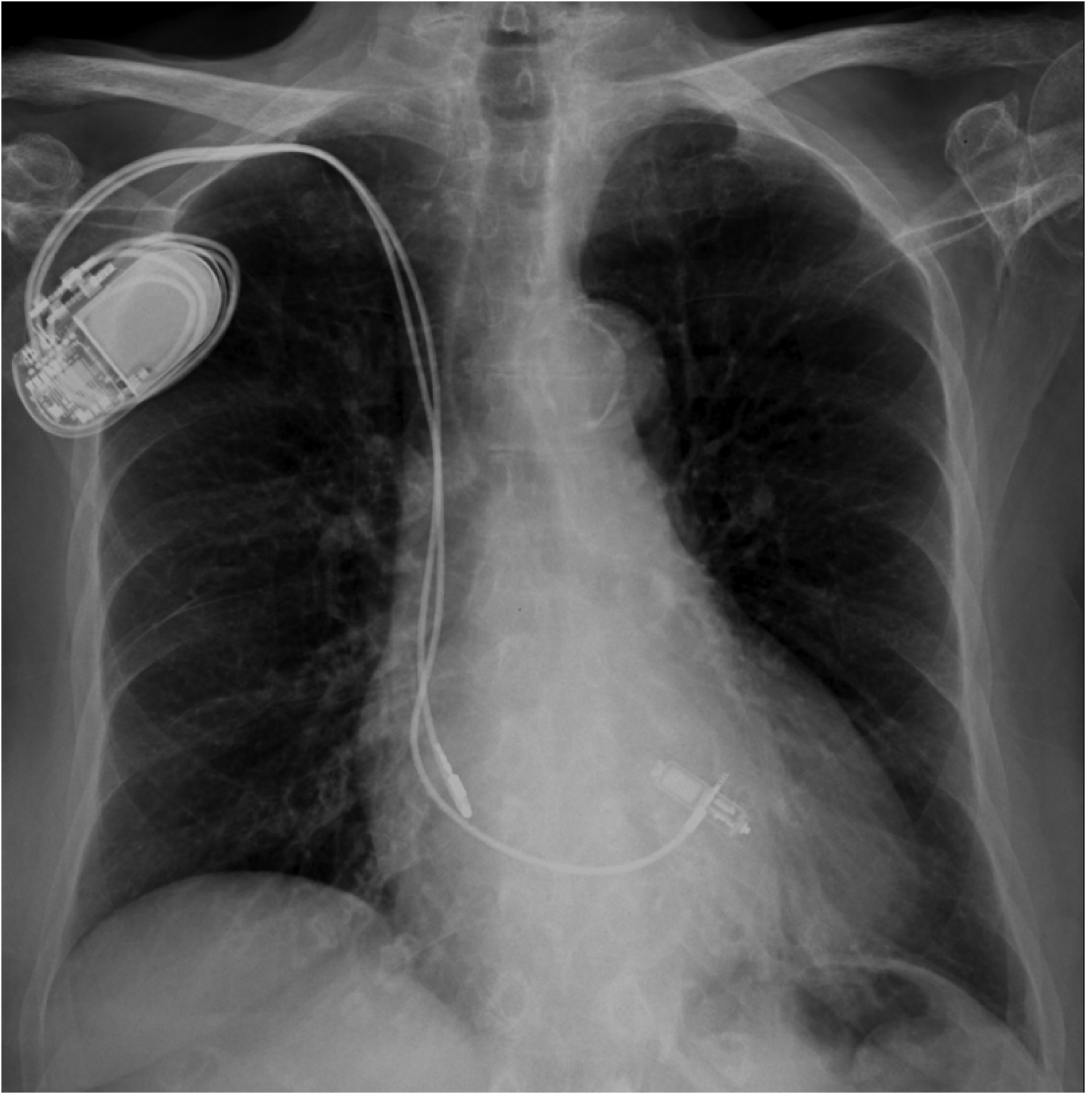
Post-procedural chest x-Ray showing a dual chamber transvenous pacemaker and a MICRA™ AV leadless pacemaker implanted at the interventricular septum.
